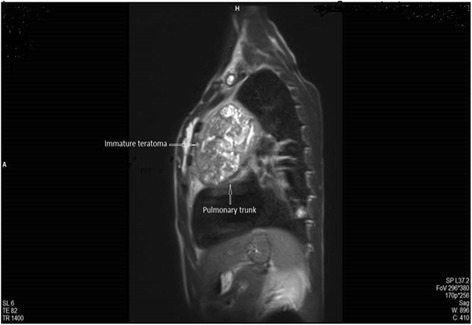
Magnetic resonance imaging showing the compression of the main pulmonary artery
